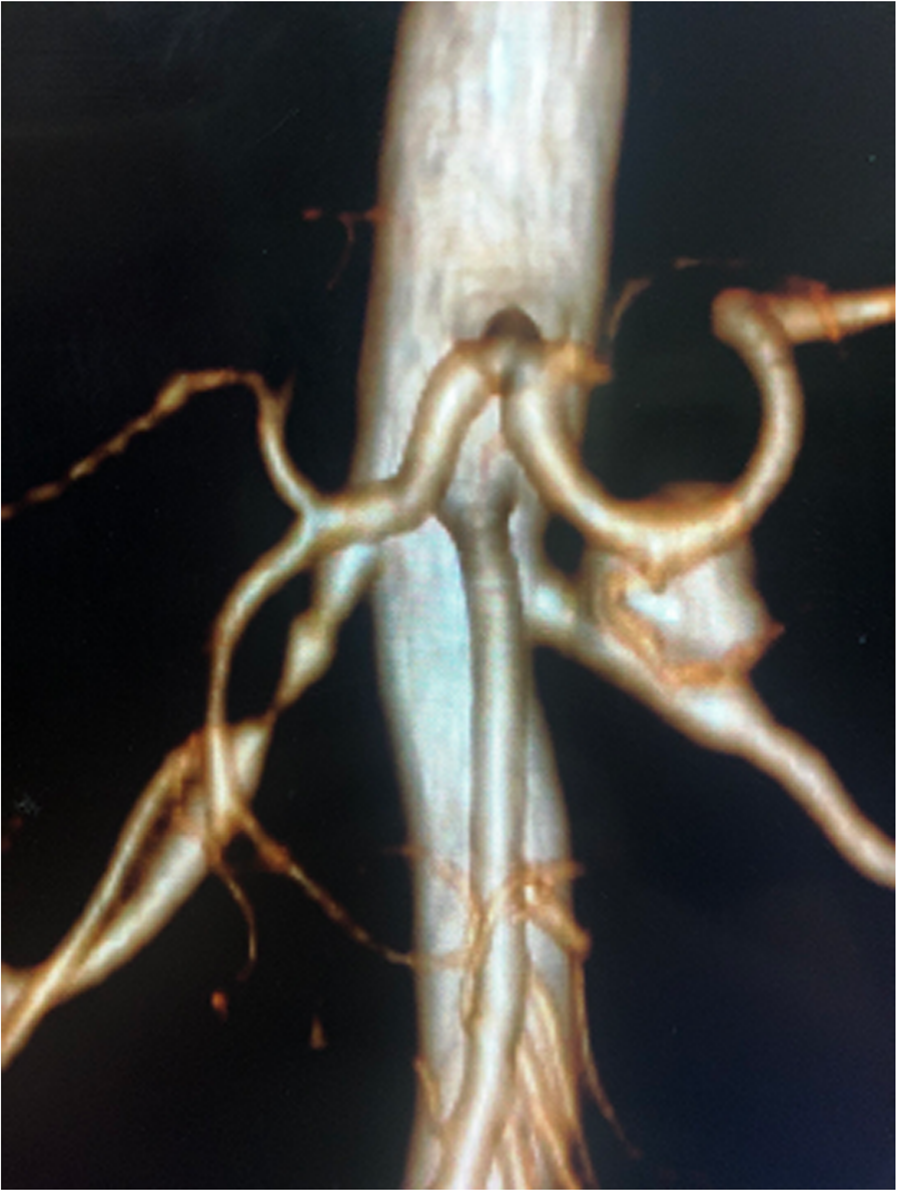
Pre-procedural Reconstructed CT showed the stenosis in the right renal artery and aneurysm in the left
